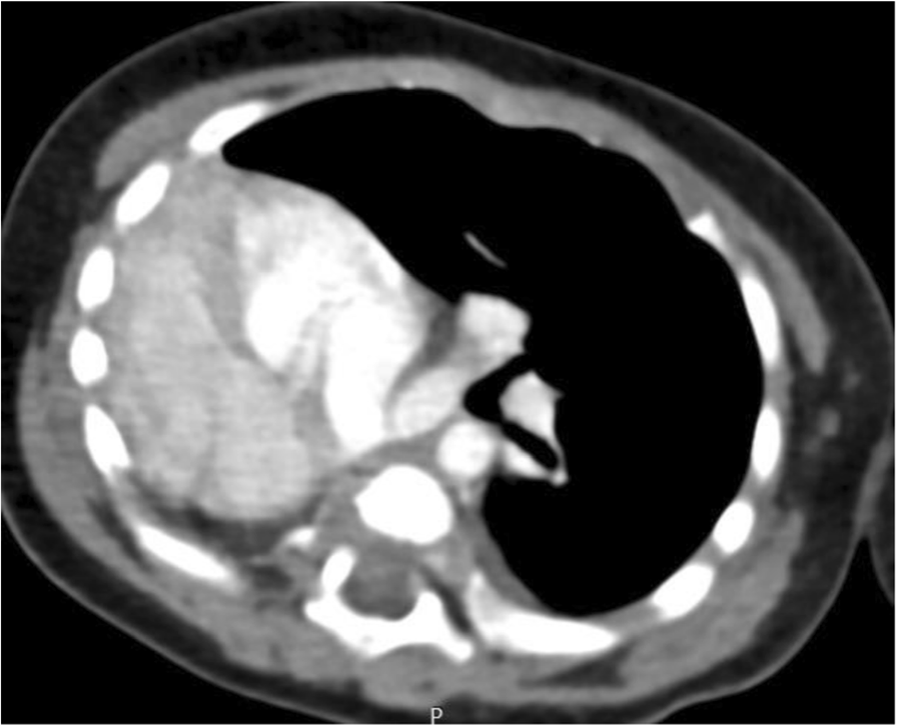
Mediastinal window CT image in axial section shows complete absent of right lung, bronchus and vessels with malposition of the heart in the right hemithorax. (Seven months’ boy)
